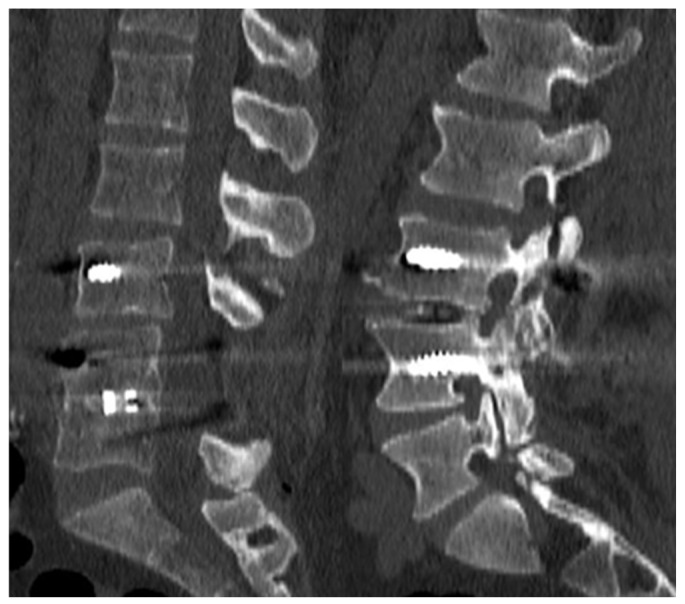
CT images of the cases with anterior and posterior fusion in the 1st year postoperatively.
